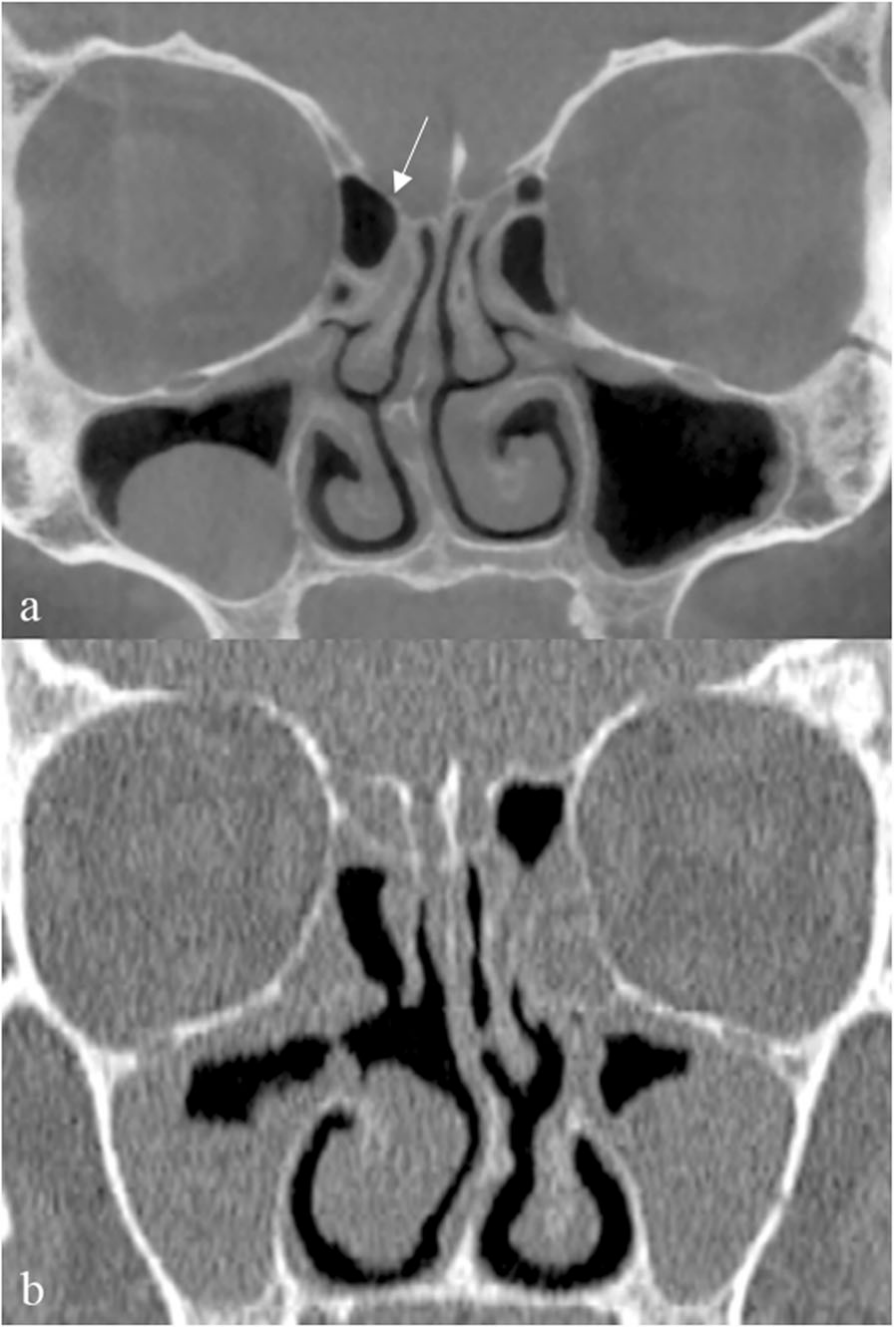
Anatomic variants of the ethmoid roof. CBCT (a) shows clear asymmetry, the slope of the right vertical lamella (arrow) being more pronounced than on the contralateral side. b MDCT shows a deep olfactory fossa (type III according to the Keros classification)
